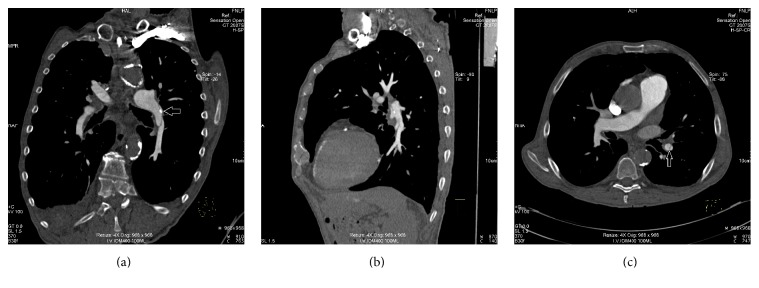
Coronal and sagittal curved MPR section (a, b) of a contrast-enhanced CT pulmonary angiogram showing long thin dissection flap in the lower lobe branch of the left pulmonary artery with partial involvement of the left pulmonary artery with a small intimal flap calcification (white arrow) and axial section with partial involvement of the lower segmental branch (white arrow) (c).
